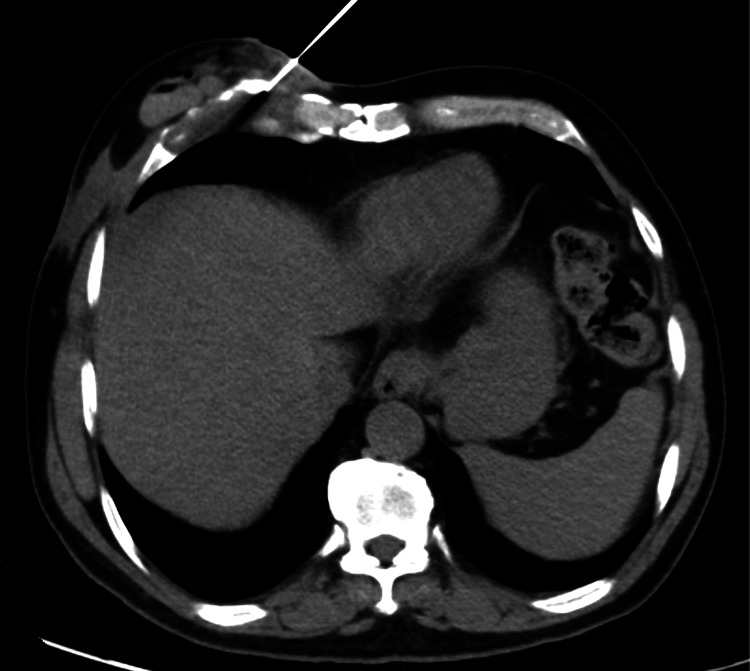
Contrast-enhanced computed tomography (CECT) guided biopsy of the right fifth costochondral junction
